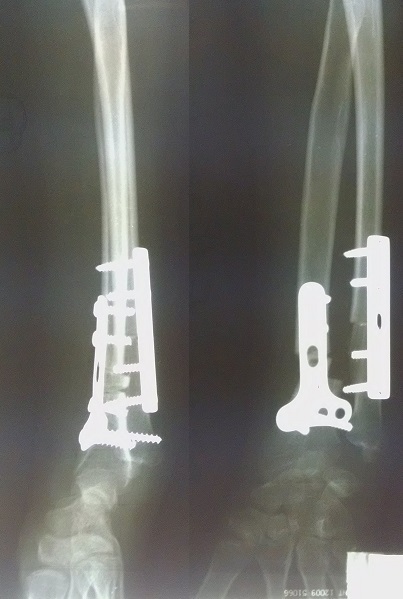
Radiographie de contrôle post opératoire immédiat
